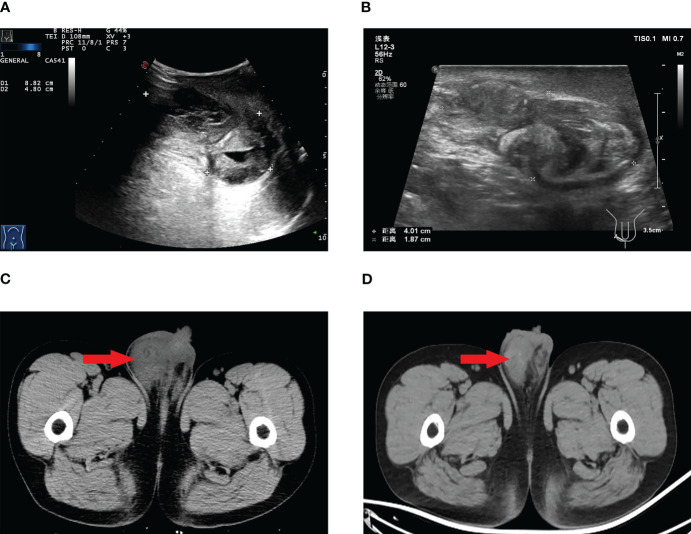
Scrotal ultrasound before targeted therapy (A), scrotal ultrasound after targeted therapy (B), scrotal CT before targeted therapy (C), scrotal CT after targeted therapy (D).
